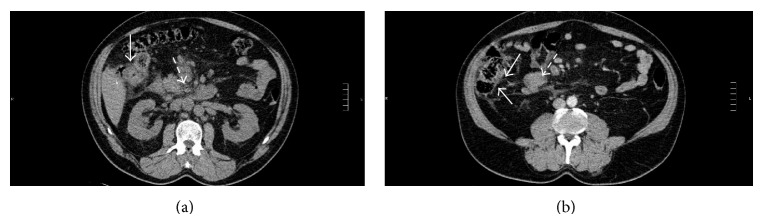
(a) Abdominal computed tomography. Circumferential thickening of the ascending colon wall (full arrow), multiple mesenteric lymphadenopathy, and thickening of the mesenteric fat (dashed arrow). (b) Abdominal computed tomography. Stranding of the pericolic fat of the ascending colon (full arrows) and lymphadenopathy conglomerate adjacent to the right anterior pararenal fascia (dashed arrow).
